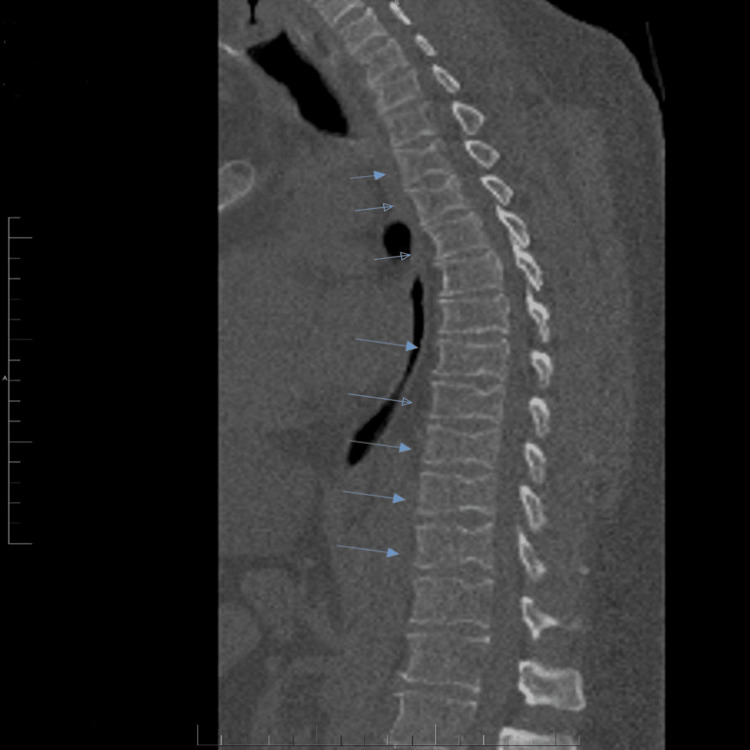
Sagittal non-contrast CT of the thoracic spine demonstrating platyspondyly (flattening of the vertebral bodies). The blue arrows indicate the affected vertebral bodies.
